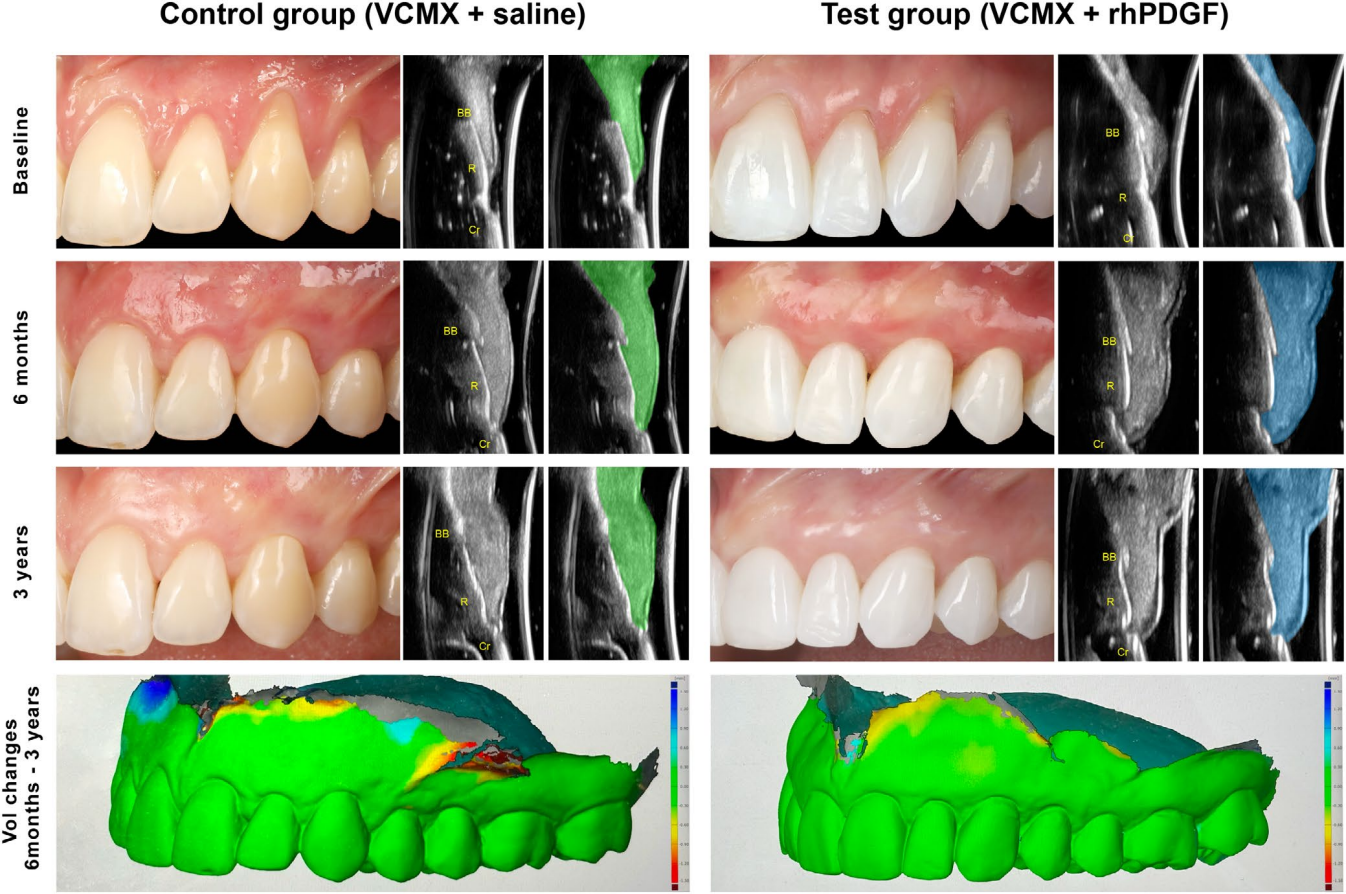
Clinical, ultrasonographic and volumetric outcomes at 6 months and 3 years following the intervention.
